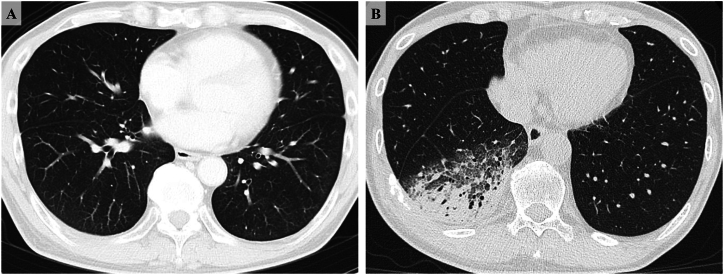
High-Resolution Computed Tomography (HRCT) Findings. (A) Baseline HRCT obtained prior to enfortumab vedotin initiation, showing no abnormal findings. (B) HRCT at presentation, revealing newly developed infiltrative shadows with air bronchograms predominantly in the right lower lobe. The peribronchovascular distribution of the infiltrates and subtle interlobular septal thickening in the periphery are consistent with an organizing pneumonia pattern.
